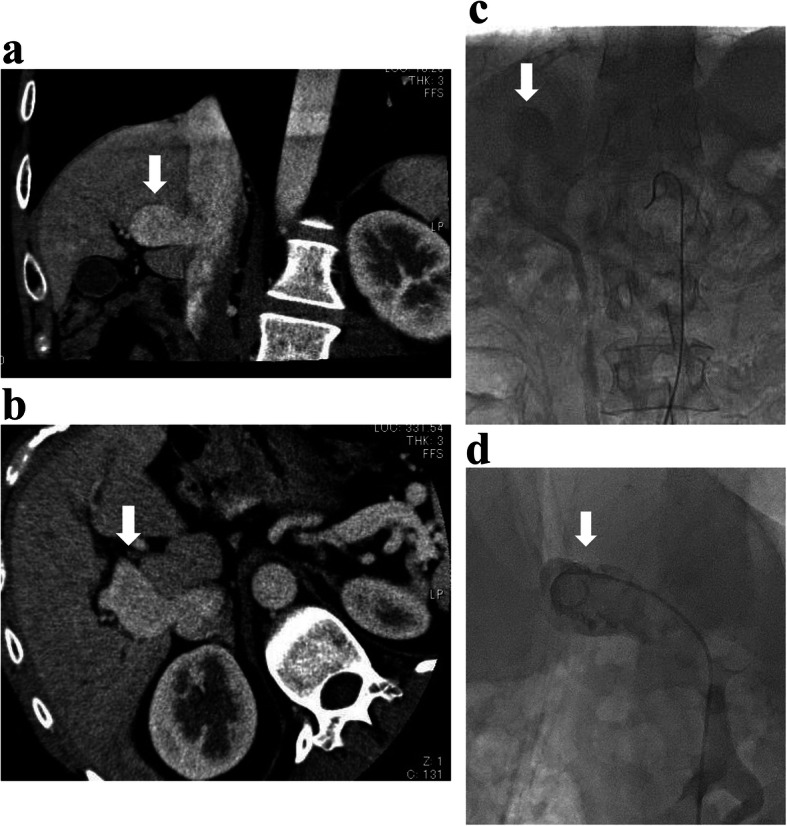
The findings of contrast-enhanced multidetector computed tomography (MDCT) and transcatheter angiogram. a, b Contrast-enhanced MDCT showing an abnormal venous connection between the left portal vein and the inferior vena cava (IVC; white arrows), suggestive of patent ductus venosus. c, d Elective superior mesenteric angiogram with delayed venous phase image and transfemoral venography showing the same findings as those of MDCT
